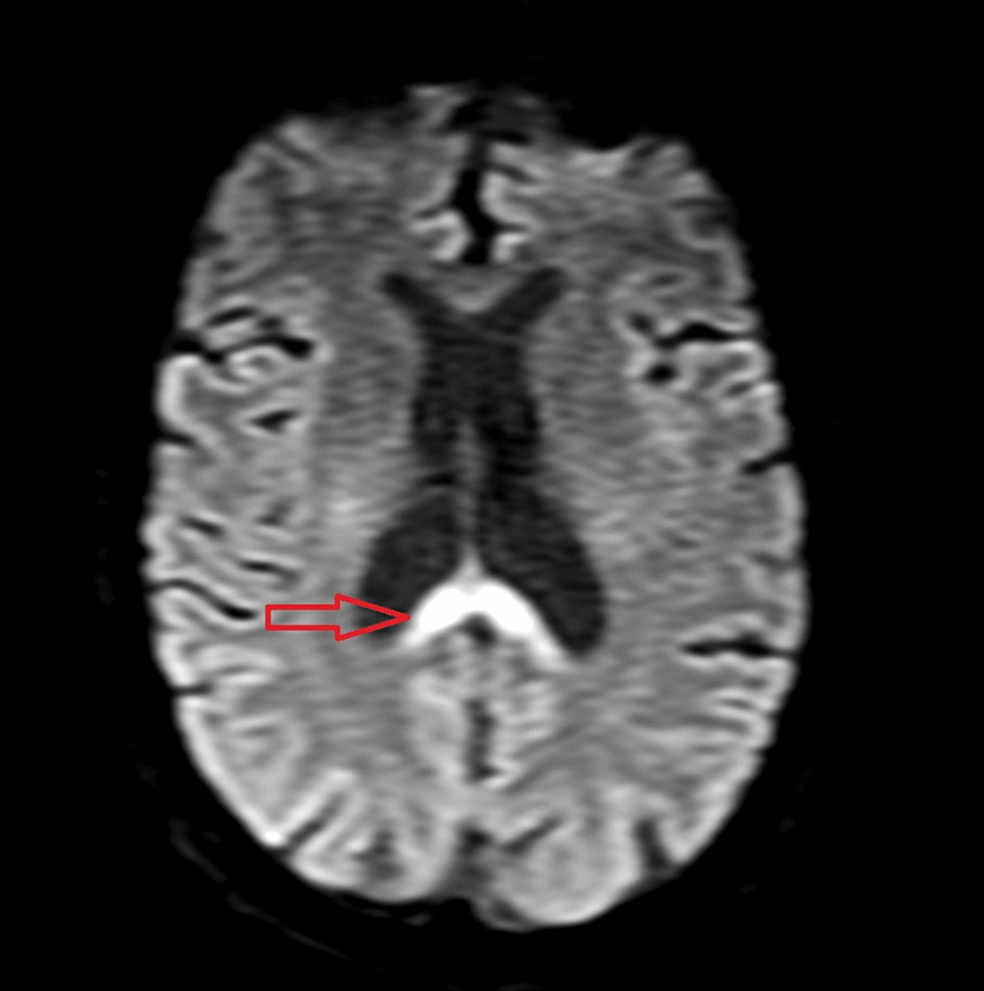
Axial DWI showing restriction to diffusion (arrow) which indicates an acute lesion. DWI: diffusion-weighted imaging
